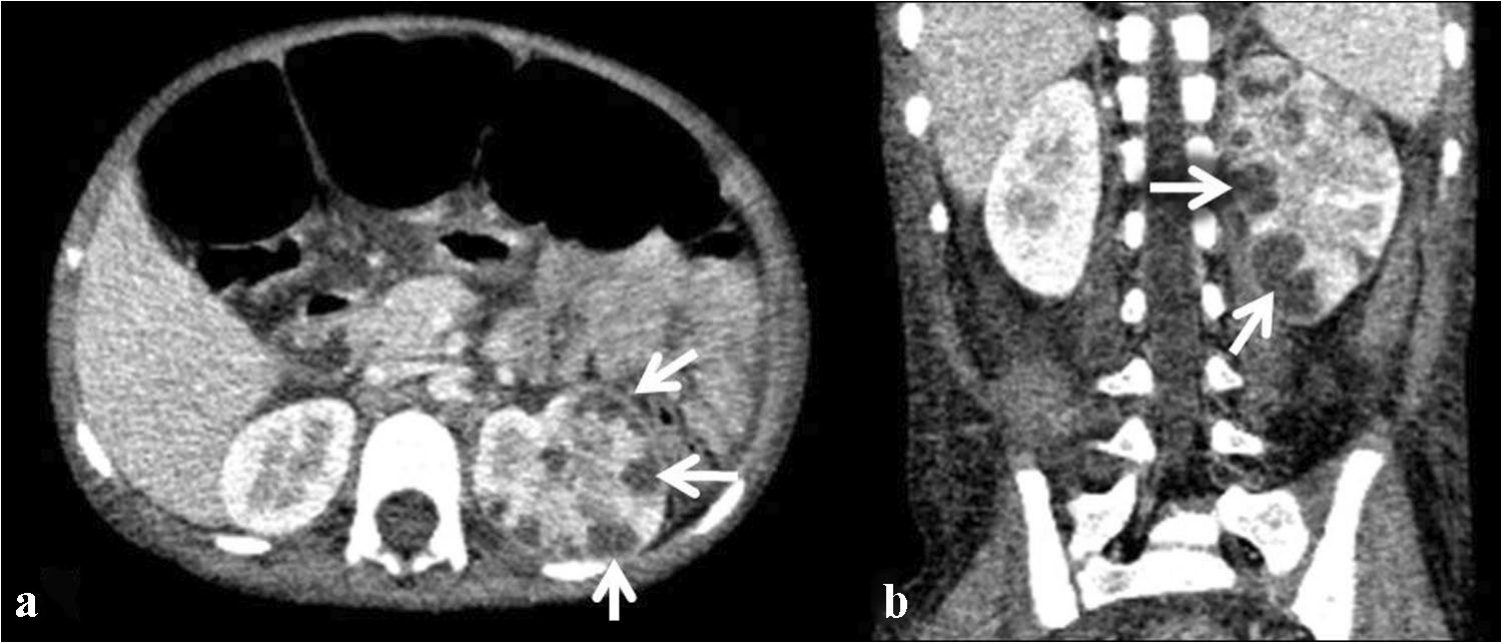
Tuberculous renal abscesses in a 5-year-old girl. Contrast-enhanced computed tomography images of the abdomen in axial (a) and coronal (b) planes show multiple peripherally enhancing hypodense lesions in the periphery of the left kidney (*arrows*)
